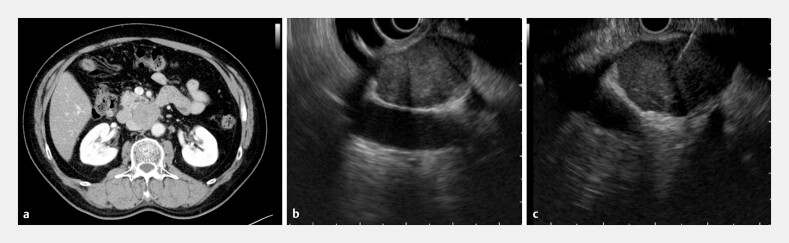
Imaging and endoscopic ultrasound (EUS)-guided fine needle aspiration (FNA; EUS-FNA) of retroperitoneal lymphadenopathy.
